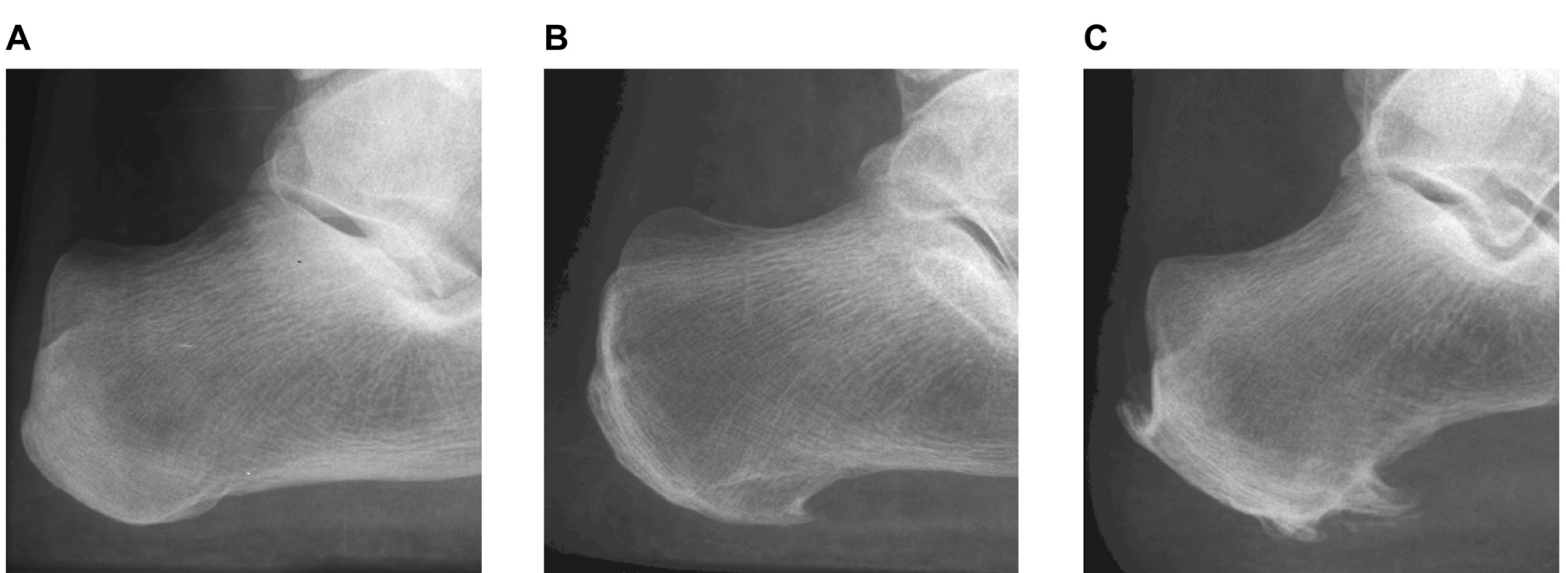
Examples of x-rays obtained in the study. A: no plantar calcaneal or Achilles tendon spur, B: plantar calcaneal spur only, C: plantar calcaneal spur and Achilles tendon spur.
